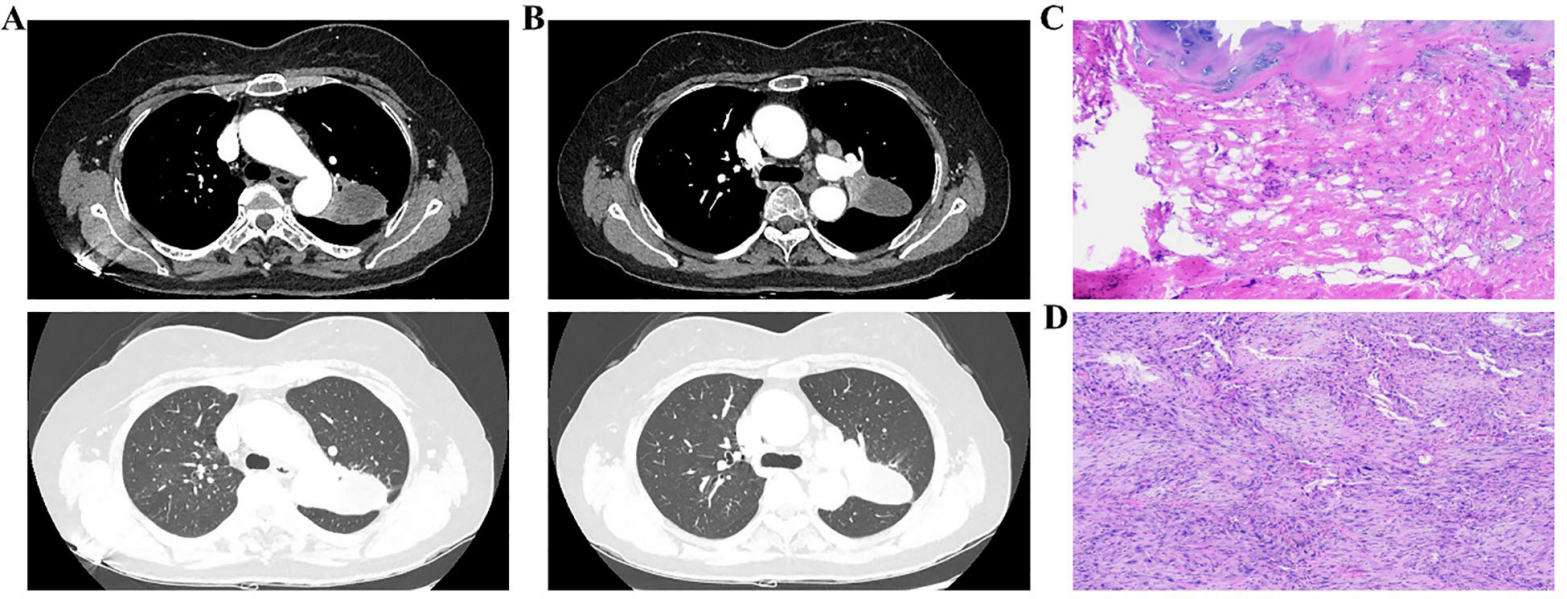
Enhanced chest CT, intraoperative bronchial margin assessment, and postoperative pathology of left upper lung lesions. (A, B) Post-treatment CT images of different facets; (C) Rapid intraoperative pathology of the bronchial margin; (D) Postoperative pathology of the left upper lobe lesion.
